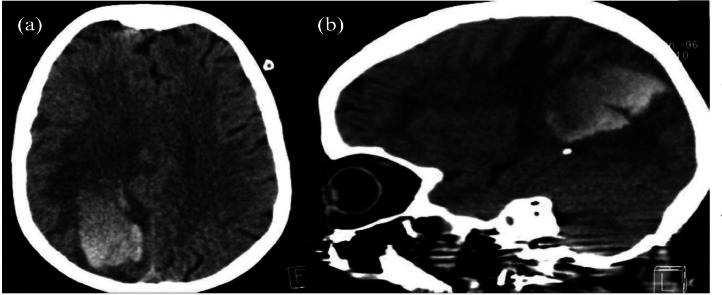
Axial (A) and sagittal (B) Nonenhanced computed tomography (NECT) of the brain showing right parieto-occipital intracerebral hematoma with blood-fluid level (arrow), associated with surrounding edema and mass effect (arrowhead).
